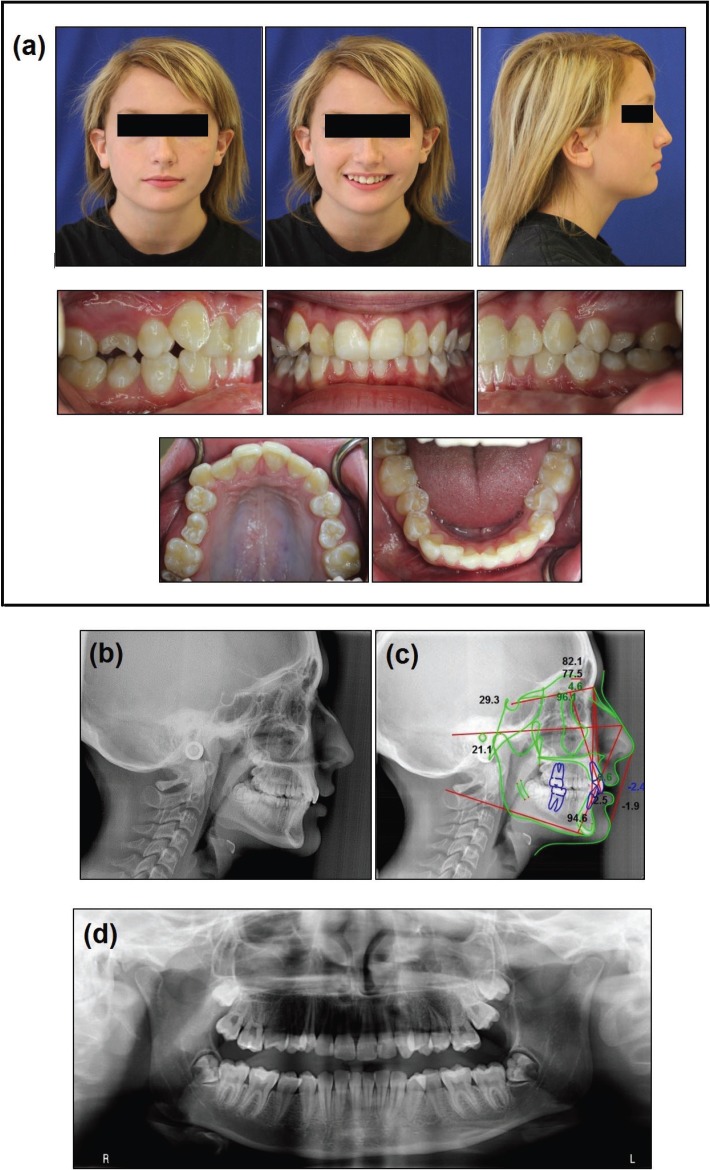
(a) Pretreatment facial and intraoral photographs, (b) Pretreatment cephalograph, (c) tracing, (d) panoramic radiograph showing opacification of left maxillary sinus region.
